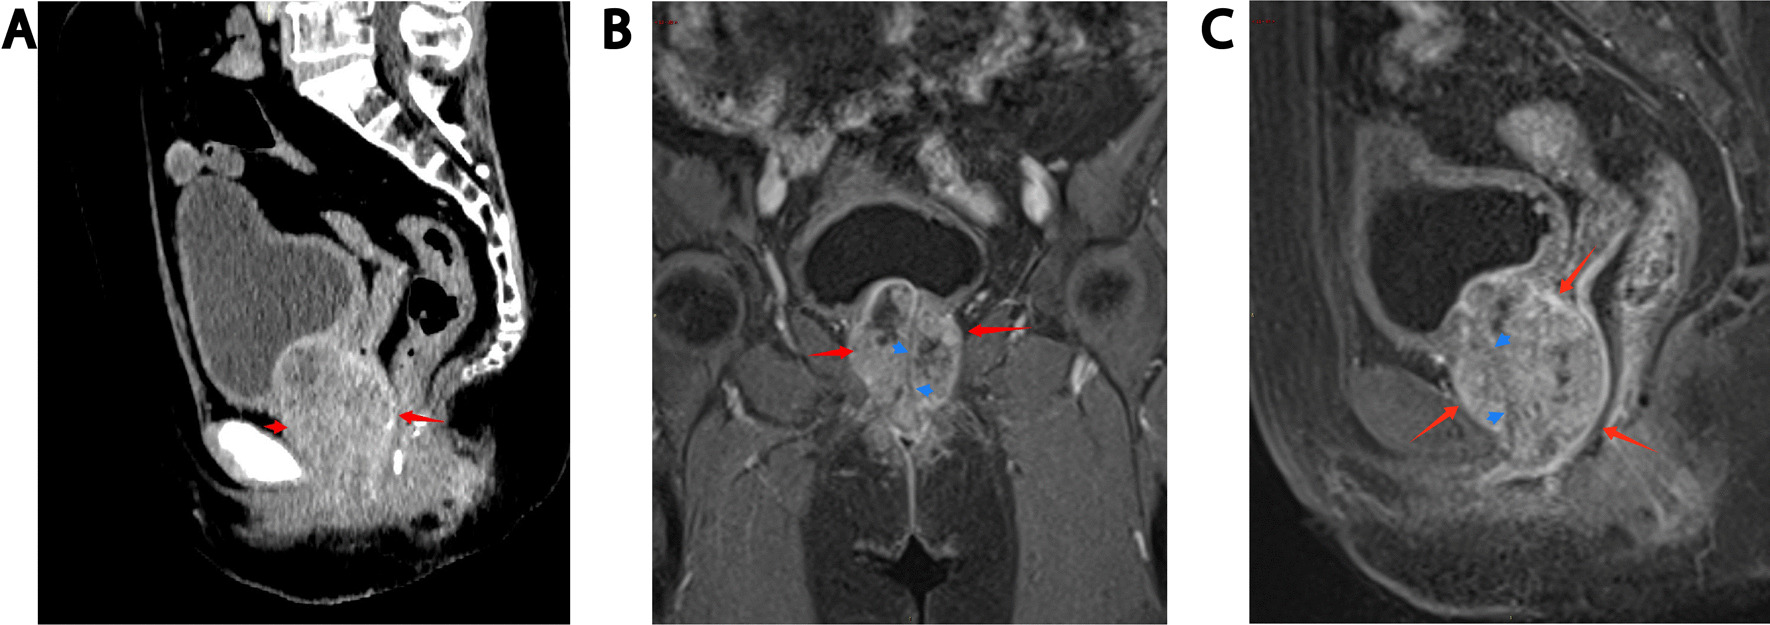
CT showed a soft tissue mass (red arrow) with a size of approximately 60*47*53 mm below the bladder and in front of the vagina (A), MRI showed a round mass (red arrrow) around the urethra with a size of approximately 45*49*62 mm, with a capsule and clear boundary, and a urethral shadow (blue arrow) could be seen in the center of the lesion (B, C)
